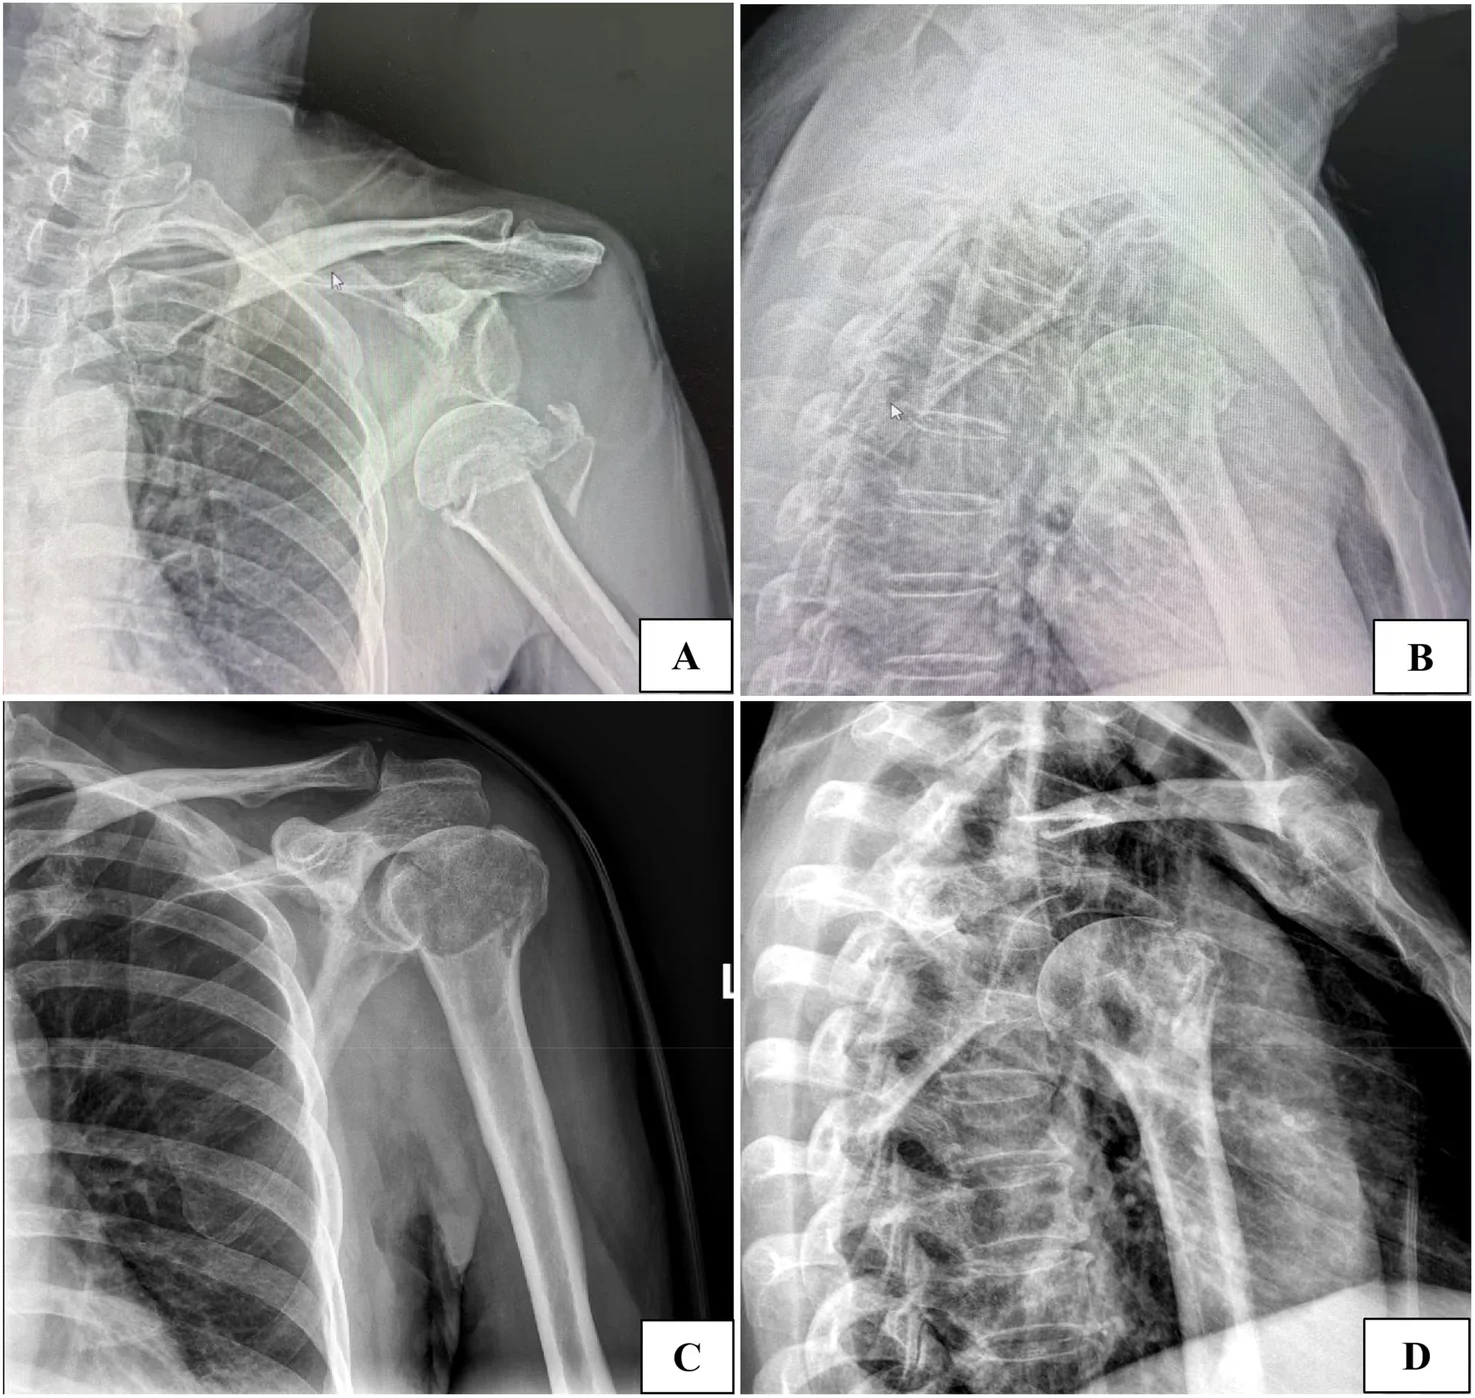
x-ray images from a 69-years-old male with anterior shoulder dislocation combined with humeral surgical neck fracture. (A,B) The x-ray shows the fracture line is transverse and belongs to type I fracture-dislocation classification. (C,D) After emergency closed reduction and external fixation, x-ray shows the dislocation has been reduced and with a good alignment of the fracture.
